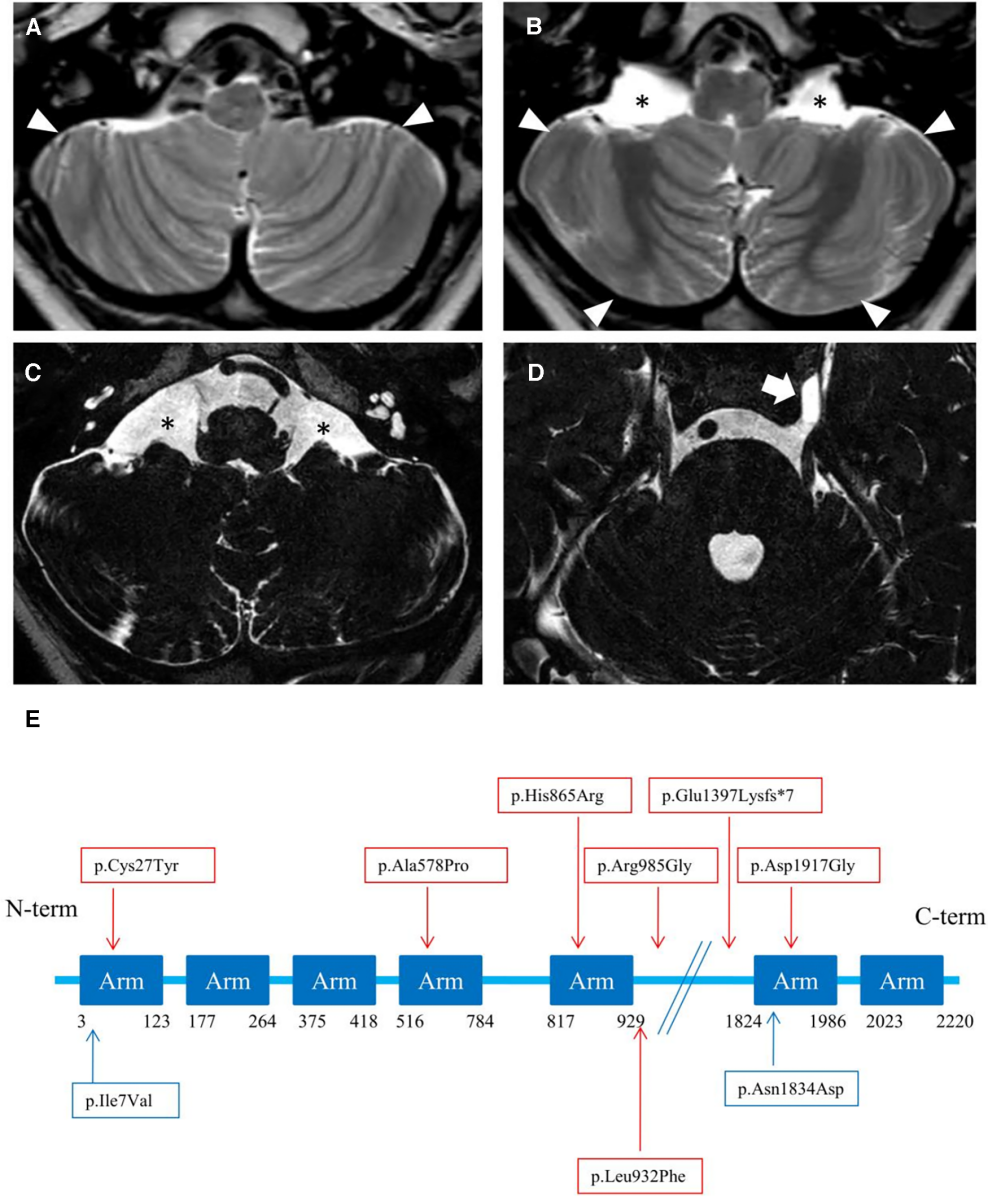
(A–D) neuroimaging of the patient. Brain MRI with axial T2-weighted images (A, B) and high-resolution heavily T2-weighted DRIVE images (C, D) reveal abnormal foliation pattern in the inferior portions of smaller cerebellar hemispheres (arrowheads). There are multiple arachnoid cysts localized in the lateral cerebello-medullary cisterns (asterisks) and left Meckel cave (thick arrow). (E) Linear structure of Rotatin protein showing Armadillo type fold (Arm aminoacids) repeat-rich areas mediating protein-protein interaction, modified from Cavallin et al. (7). The variants identified in the patient of this study are highlighted in blue, others are reported in red.
